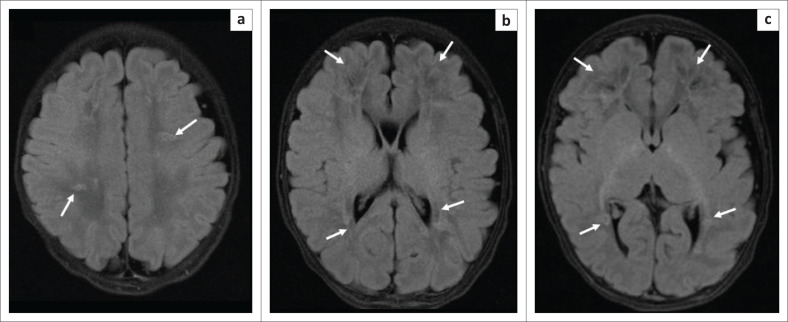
Axial fluid-attenuated inversion recovery MRI of the brain (inversion time 2500, repetition time msec/echo time msec, 9000/112, 3-mm section thickness) at the level of the high frontal lobes (a), basal ganglia (b) and peritrigonal white matter (c). Images demonstrate areas of FLAIR signal abnormality involving the corpus callosum and the bilateral supratentorial white matter, predominantly the bilateral frontal regions (solid white arrows on all images) with sparing of the thalami.
